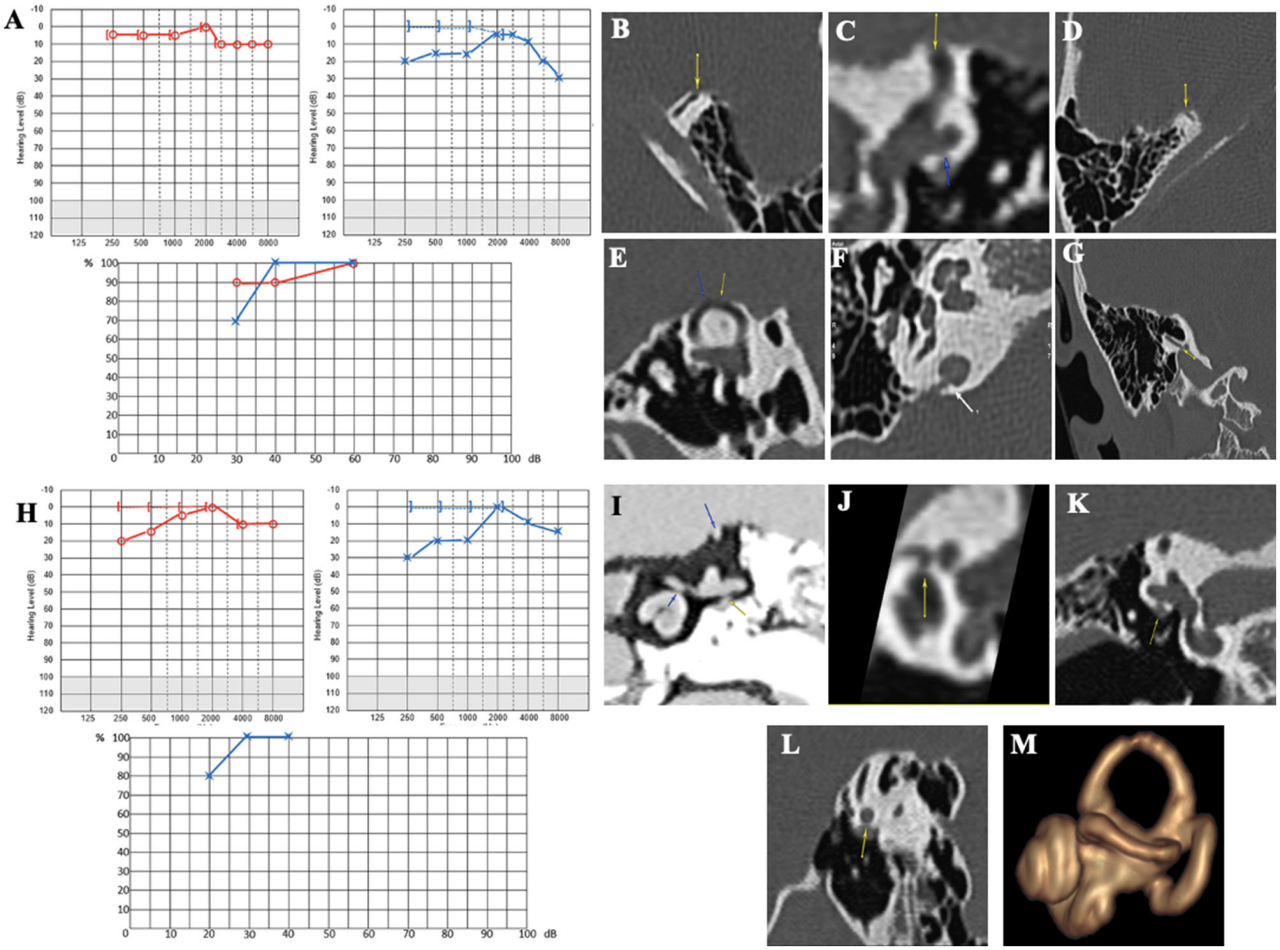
(A) Tonal and vocal audiometry showing a mild conductive hearing loss on the left side. (B, C) Left SDC in axial (B) and coronal plane (C). (D, E) Right SDC in axial (D) Pöschl plane (E). (F, G) Dehiscence between right IVJ and vestibular aqueduct in axial (F) and coronal plane (G). (H) Post-operative tonal and vocal audiometry. (I) Coronal plane in negative contrast showing near-cochleofacial dehiscence, near-dehiscence between LSC and FN II and SSCD. (J) Near-cochleofacial dehiscence. (K, L) Near-cochleofacial between the right LSC and FN II in coronal (K) and sagittal plane (L). (M) Post-operative 3D MRI showing an effective capping of SCC.
